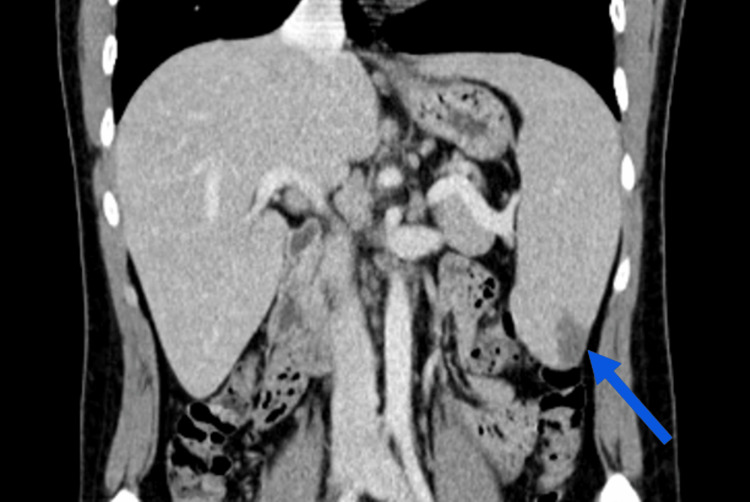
Coronal contrast-enhanced chest and abdominopelvic computed tomography showing a hypodense area on the posteroinferior aspect of the spleen (splenic infarction; blue arrow)
